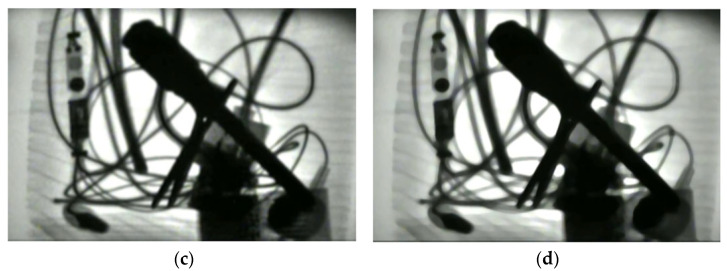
Image fuzzy of prohibited items X-ray image. (a) The original captured X-ray image; (b) The image with Gaussian blurring; (c) the image with averaging blurring; (d) the image with median blurring.
